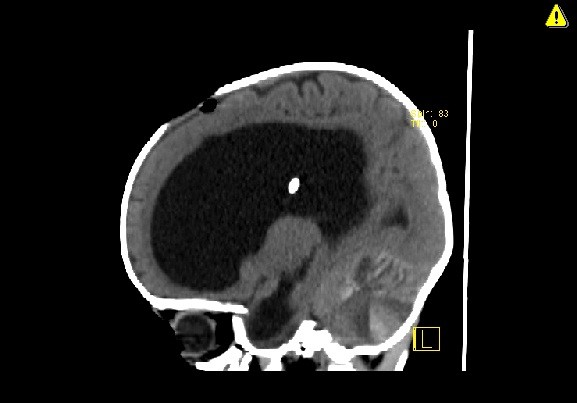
Sagittal computed tomography scan demonstrating the extent of the subcortical and intraparenchymal cerebellar hemorrhage. Note the classic zebra sign.
